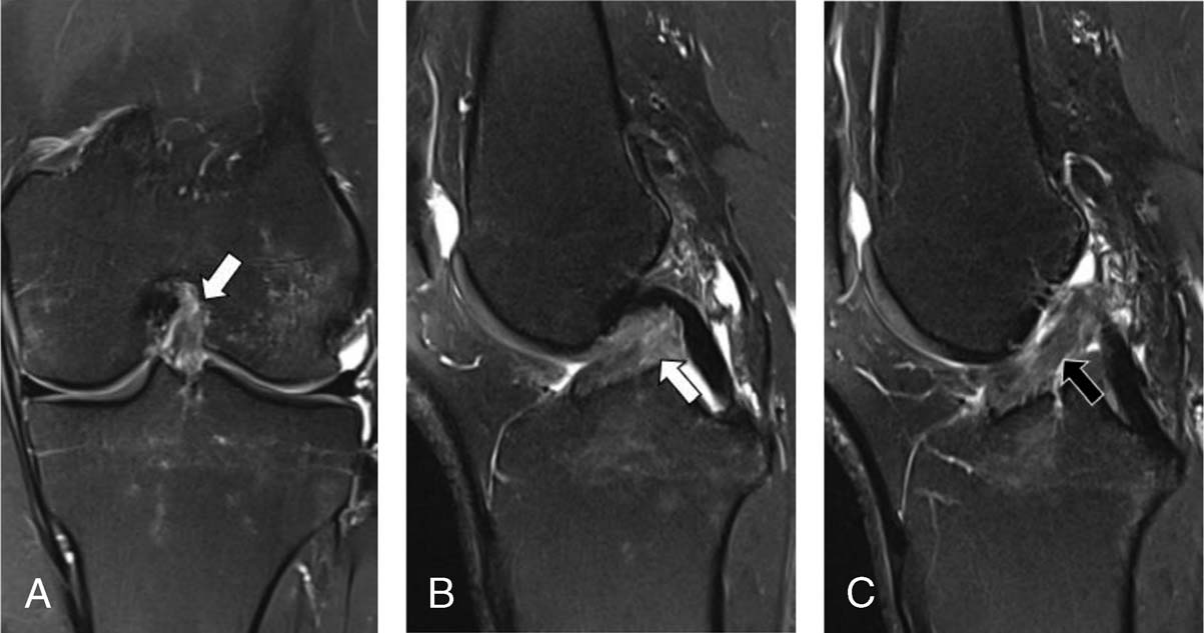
MRI of the left knee of a 38-year-old woman with knee injury 3 months earlier. Coronal intermediate-weighted turbo spin echo MRI scan with fat suppression (A) and sagittal intermediate-weighted turbo spin echo MRI scans with fat suppression (B and C) show tearing of the anterior cruciate ligament with greater than 80% disruption of fibers (white arrows) and some intact fibers (black arrow) remaining, as confirmed by surgery. Two of the 3 radiologists classified the MRI scans as a partial-thickness tear (<80% of fiber discontinuity), representing a false-negative interpretation. One radiologist and the DCNN correctly diagnosed a full-thickness ACL tear, representing a true-positive interpretation.
